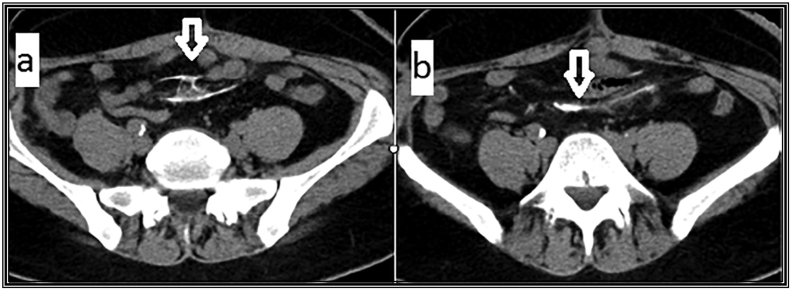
Abdominal computerized tomography (CT) with the contrast. (a) Axial view of the mesentery shows irregular dense calcified shadows (white arrow) not connected to the adjacent bowel surface. (b) Axial view shows ill-defined diffuse fat stranding opacities (white arrow).
